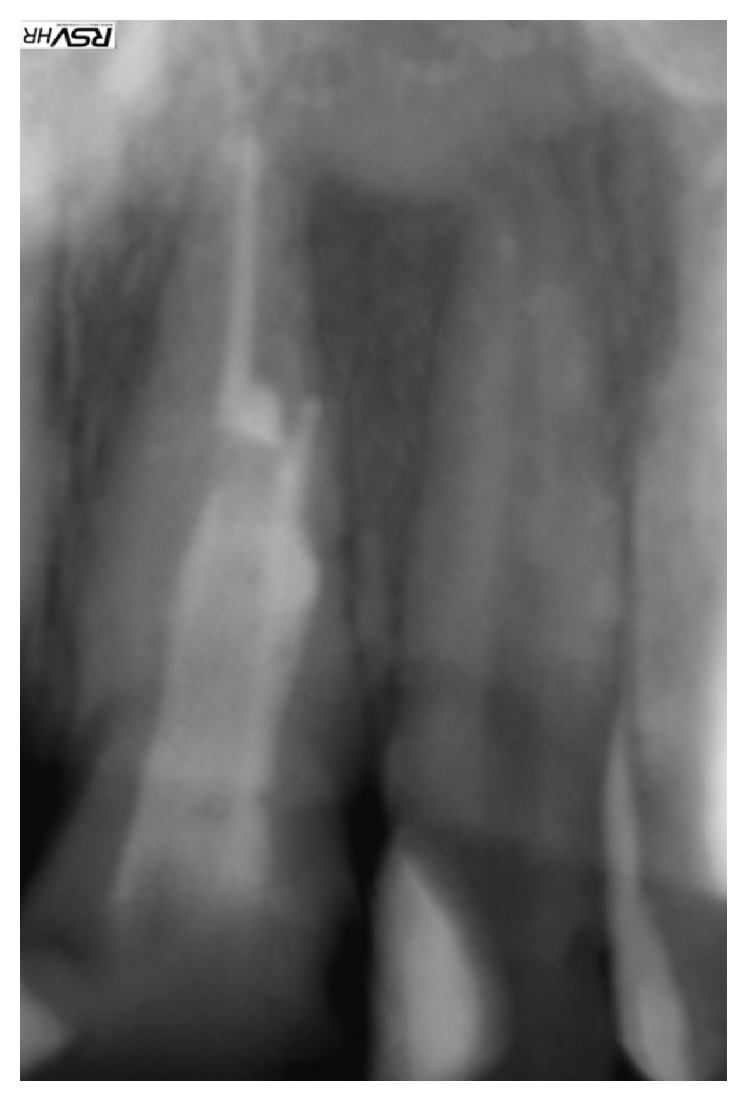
Radiograph at 1-year follow-up.
